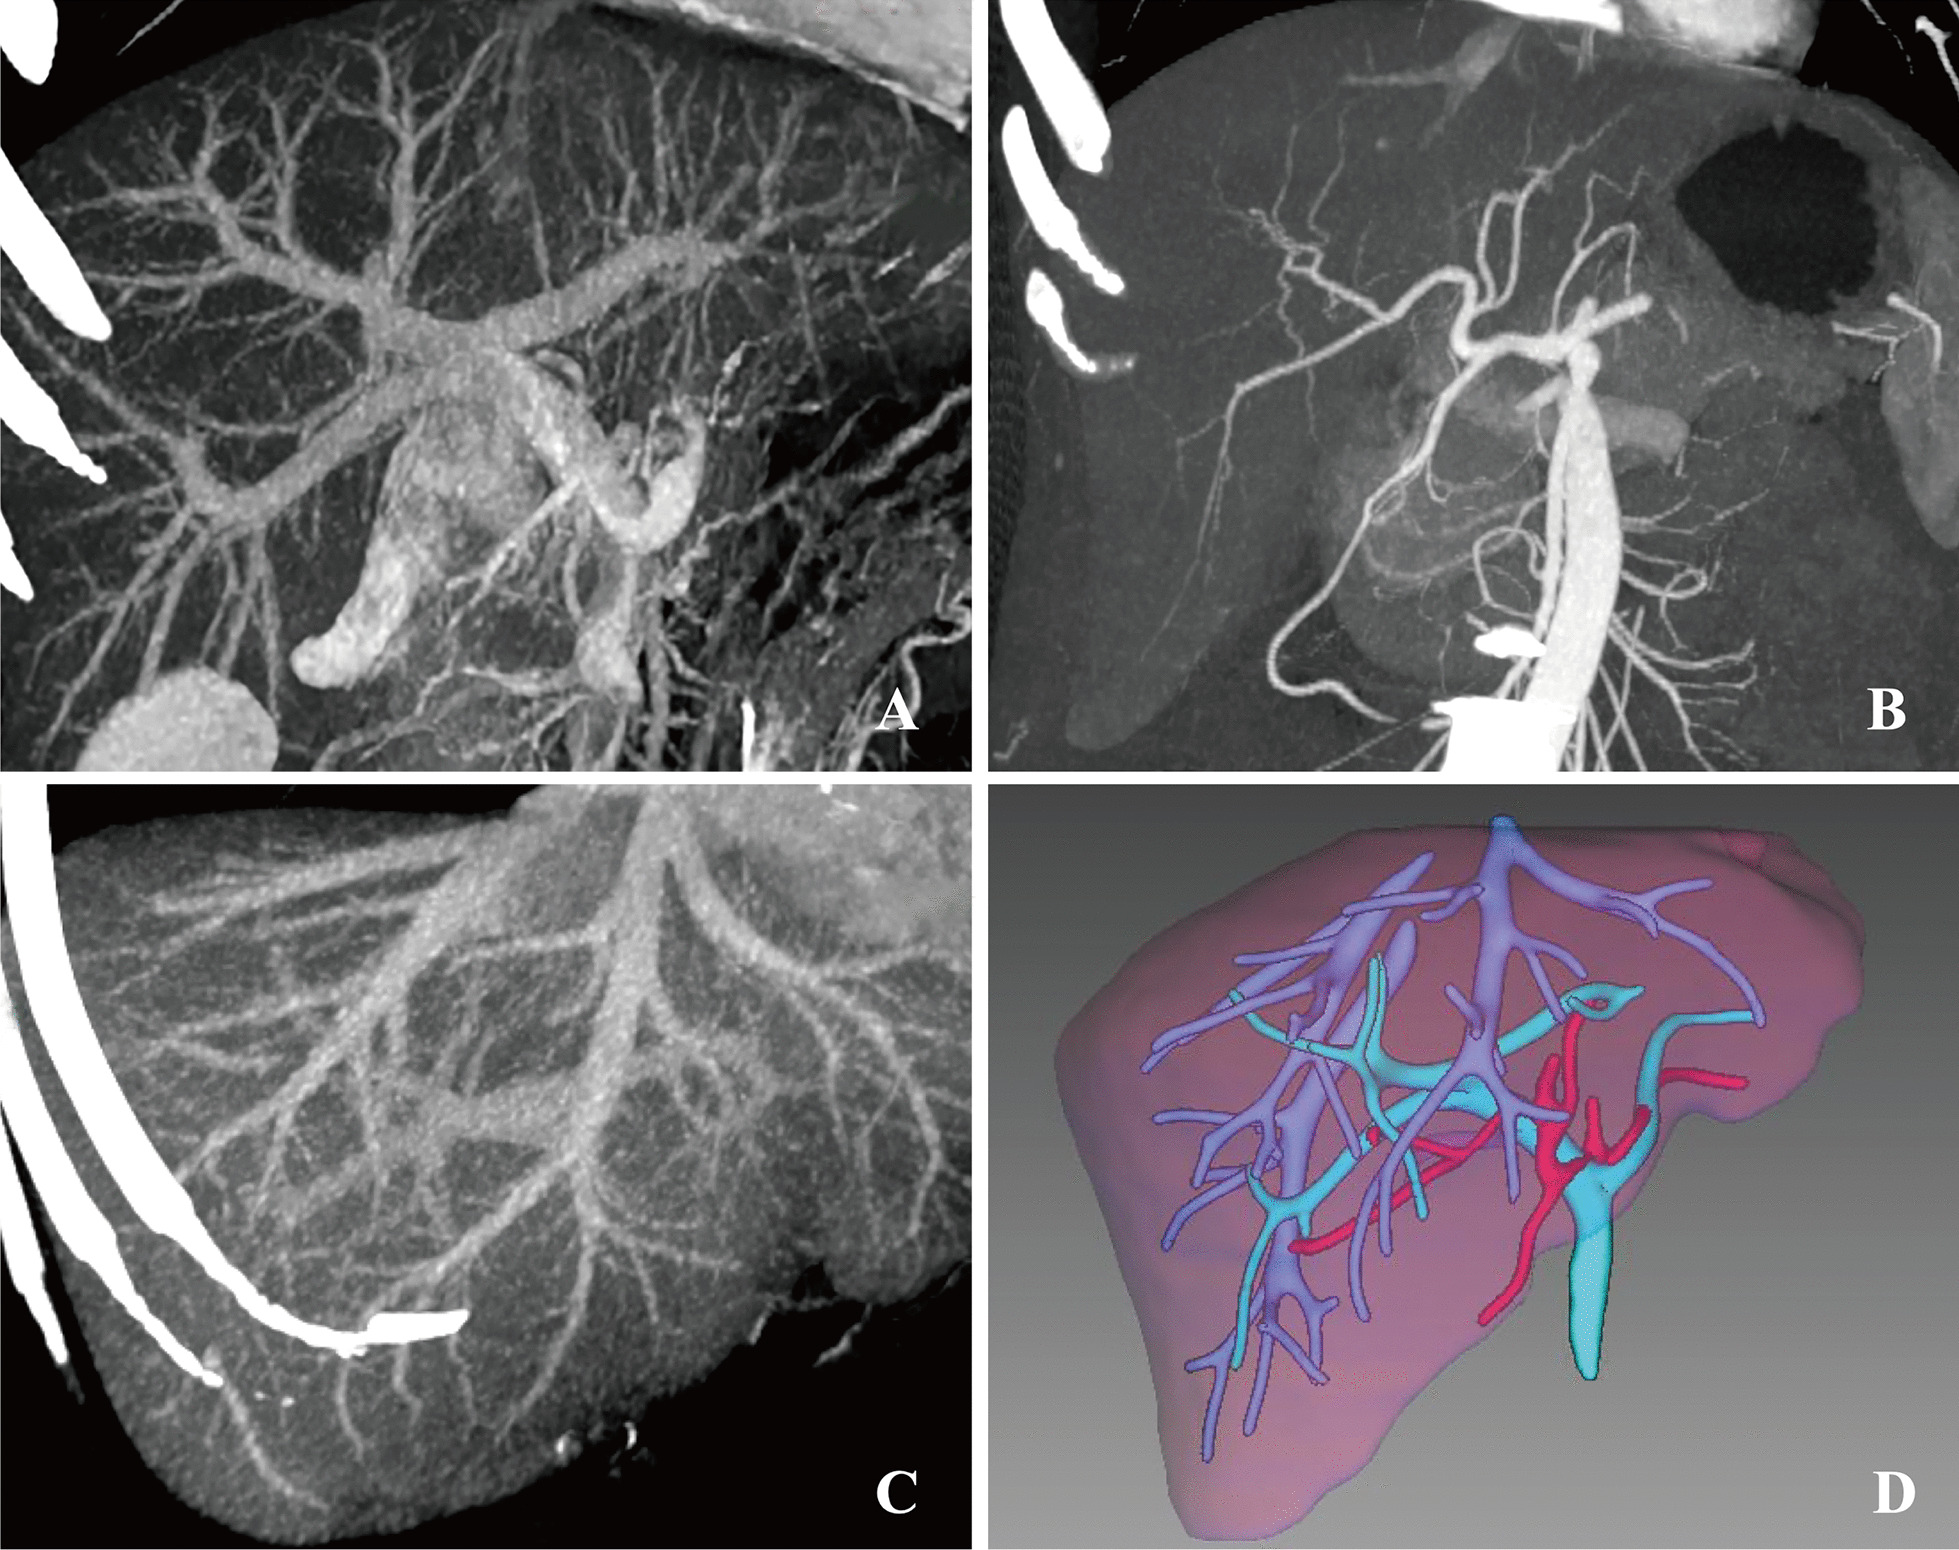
Donor’s preoperative CT 3-dimensional reconstruction of anatomical variations of case 1. A The Posterior right branch branched off separately from the main portal vein, which was classified as Type-III portal vein. B The right posterior hepatic artery branched extrahepatically. C The outflow system. D Three-dimensional imaging of hepatic blood vessels
